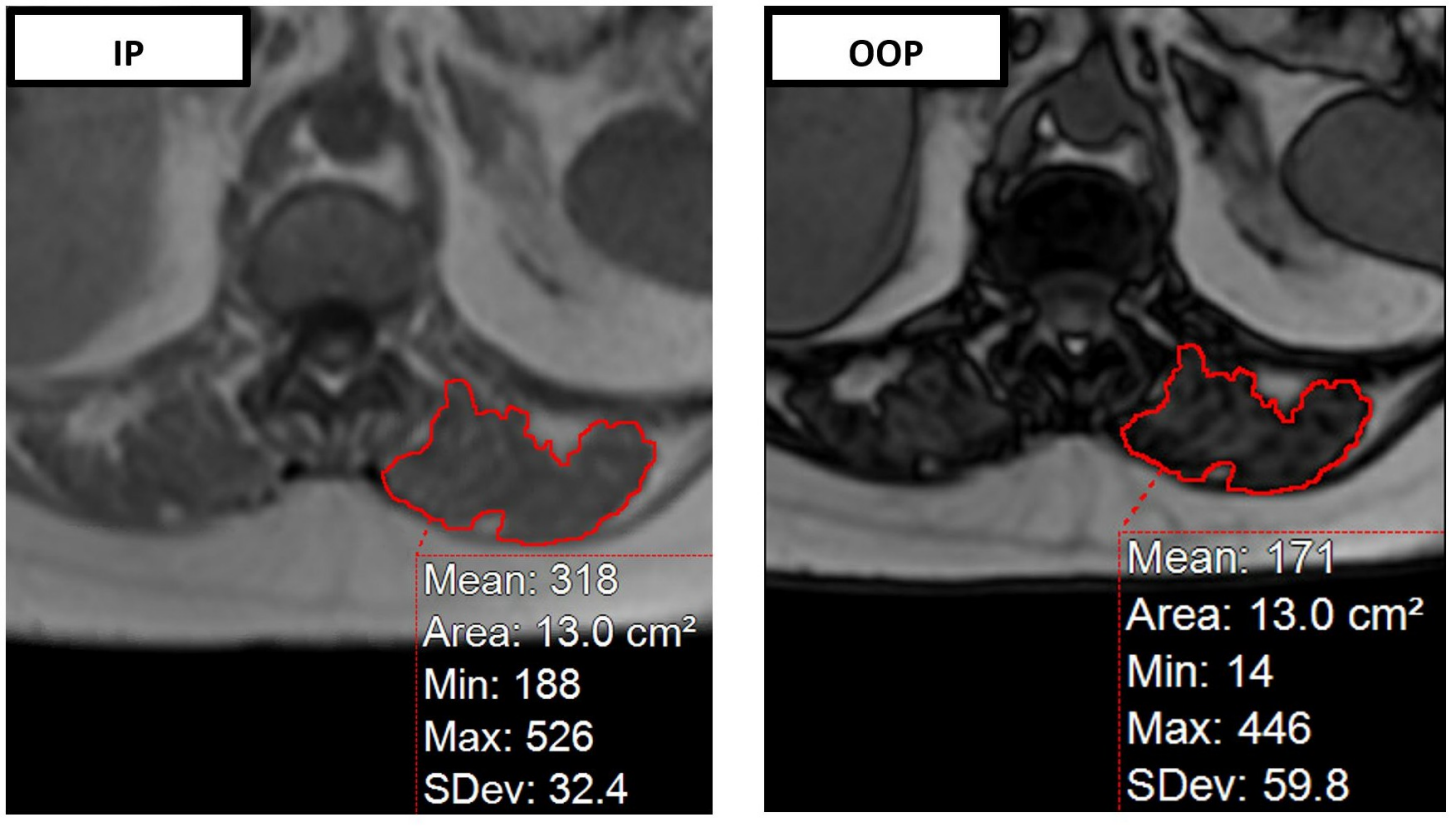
Segmentation of paraspinous muscles to calculate SMI and sFF. Segmentation of paraspinous musculature on in-phase (IP) (left) and out of phase (OOP) (right) paired MRI gradient echo (GRE) sequences at the level of the superior mesenteric artery origin.
